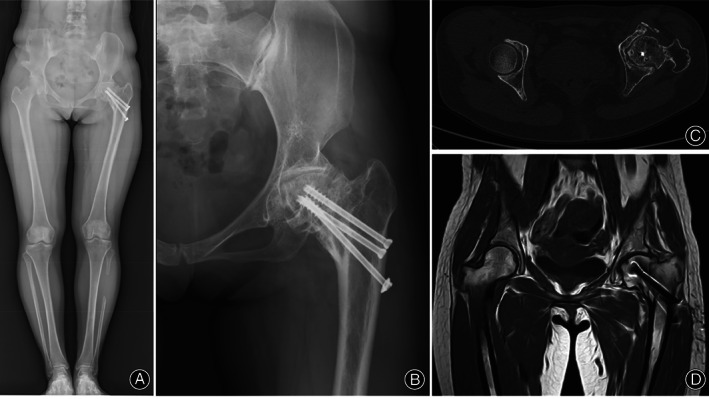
The total length of the lower limbs of the patient (A), X‐ray (B), CT (C), and MRI (D) of the patient's femoral neck 7 years after surgery.
